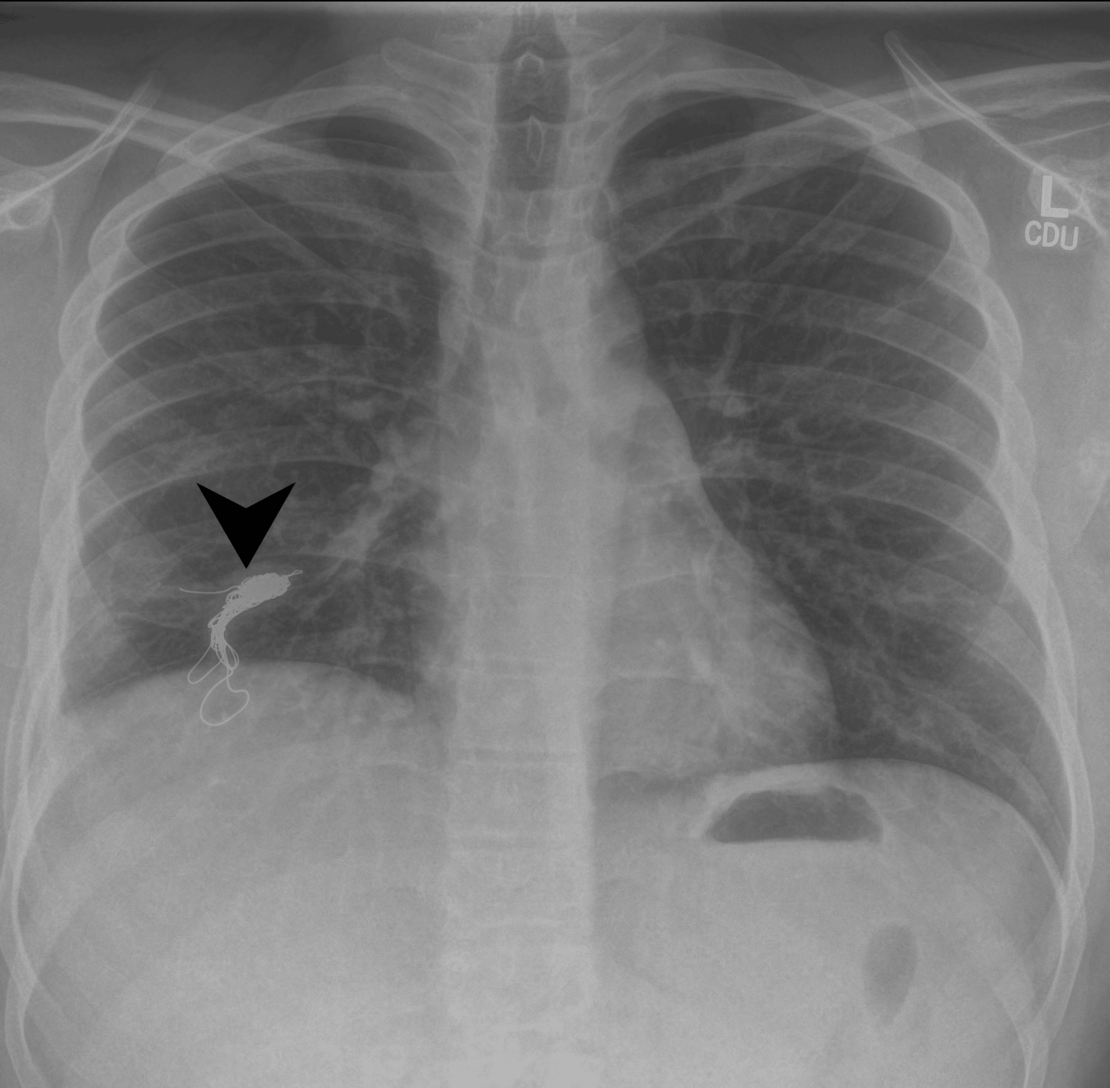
Frontal chest radiograph showing an embolization coil projected over the right lower zone (arrowhead). This finding is consistent with prior treatment of a Rasmussen aneurysm. The lungs and pleural spaces are grossly clear, with no acute abnormalities.
